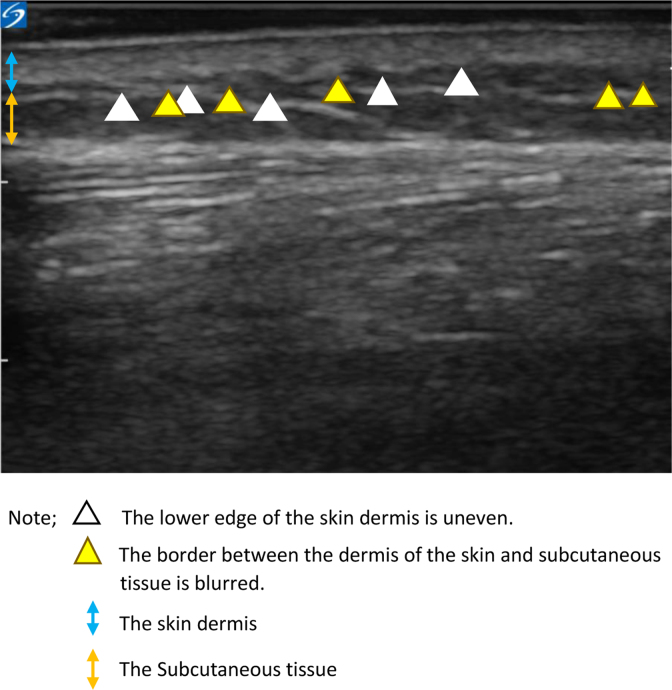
Initial findings of lower limb edema with docetaxel of ultrasonic images of 15 cm below the fibula head.
